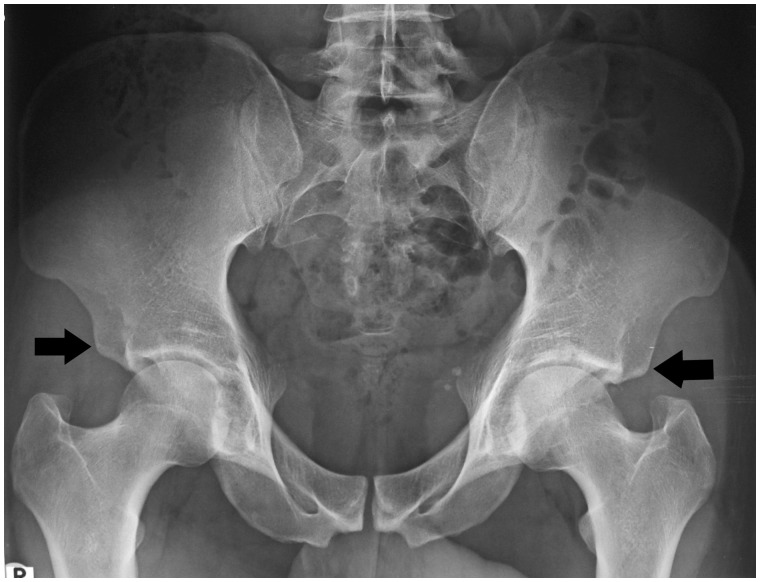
Anteroposterior plain radiograph of a 30-year-old male recreational football player. The AIISs (arrows) are at the level of (right hip) and below (left hip) the acetabular rim. Note the concurrent positive crossover signs and prominent ischial spines, suggesting apical retroversion of the acetabulae.
